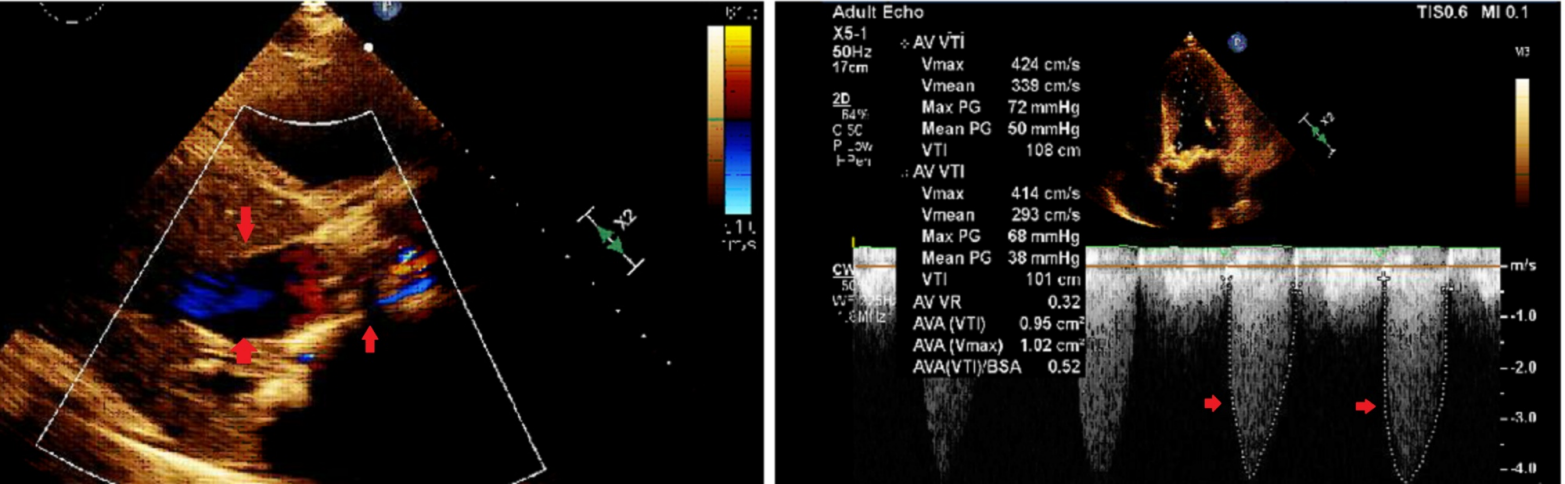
Transthoracic echocardiogram with severe aortic stenosis and elevated gradients across the valve
